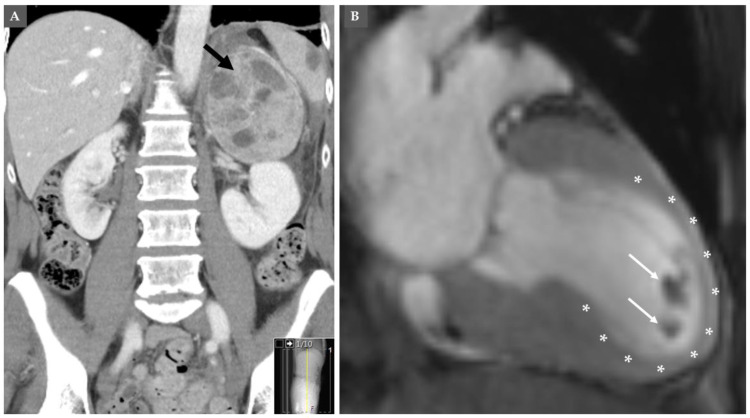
Computed tomography (CT) of the abdomen shows left-sided pheochromocytoma shown in (A) (black arrow). This pheochromocytoma has induced mid-apical pattern of takosubo syndrome (asterix), which was complicated by left ventricular thrombus at the apical region of the left ventricle (white arrows) as shown by cardiac magnetic resonance imaging in (B).
